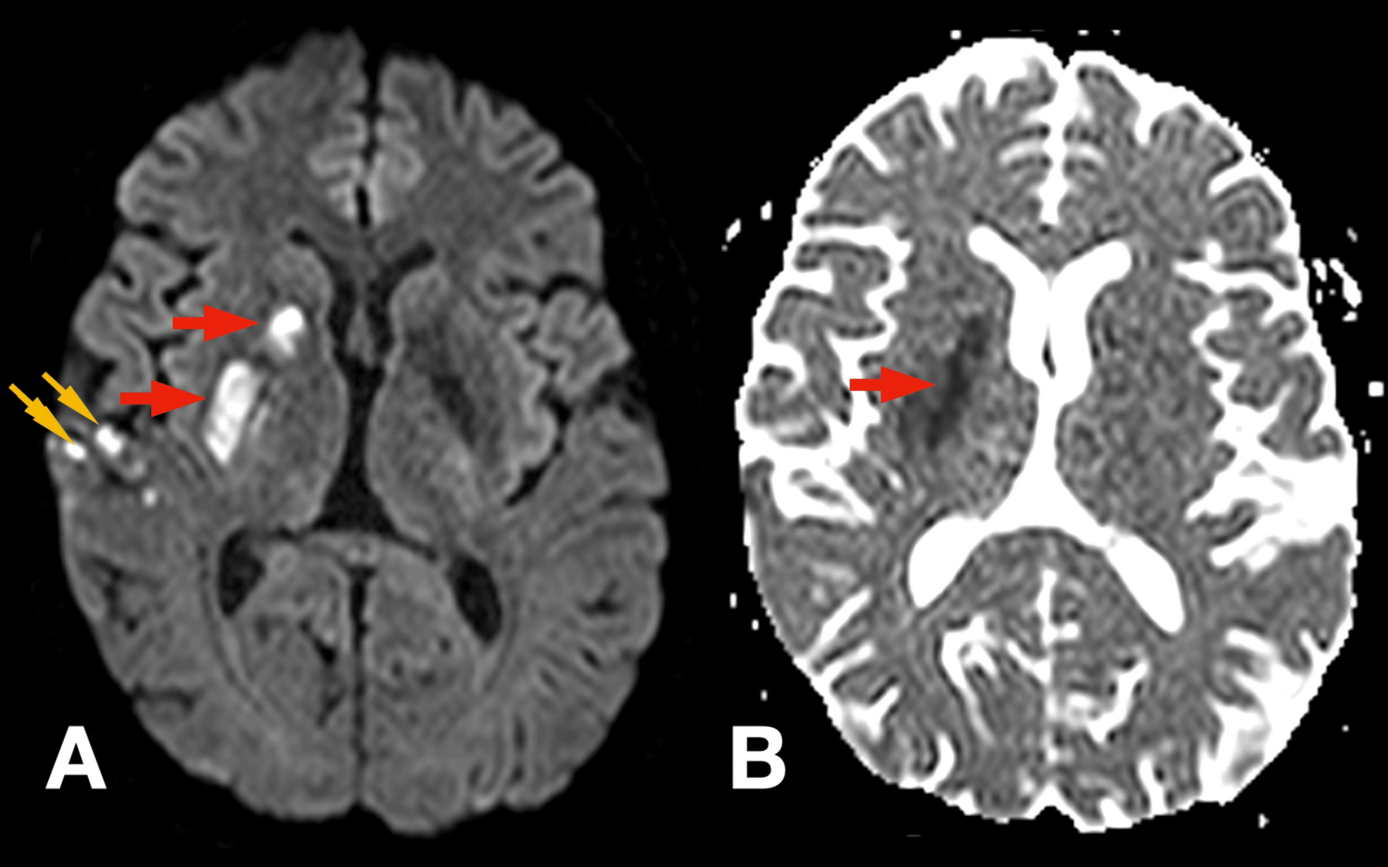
Cranial MRI demonstrating multi-focal ischemic stroke The lesions appear as hyperintense areas on diffusion-weighted image (DWI) (Panel A) and as correlative hypointense areas on apparent diffusion coefficient (ADC) (Panel B), in a pattern of restricted diffusion.
